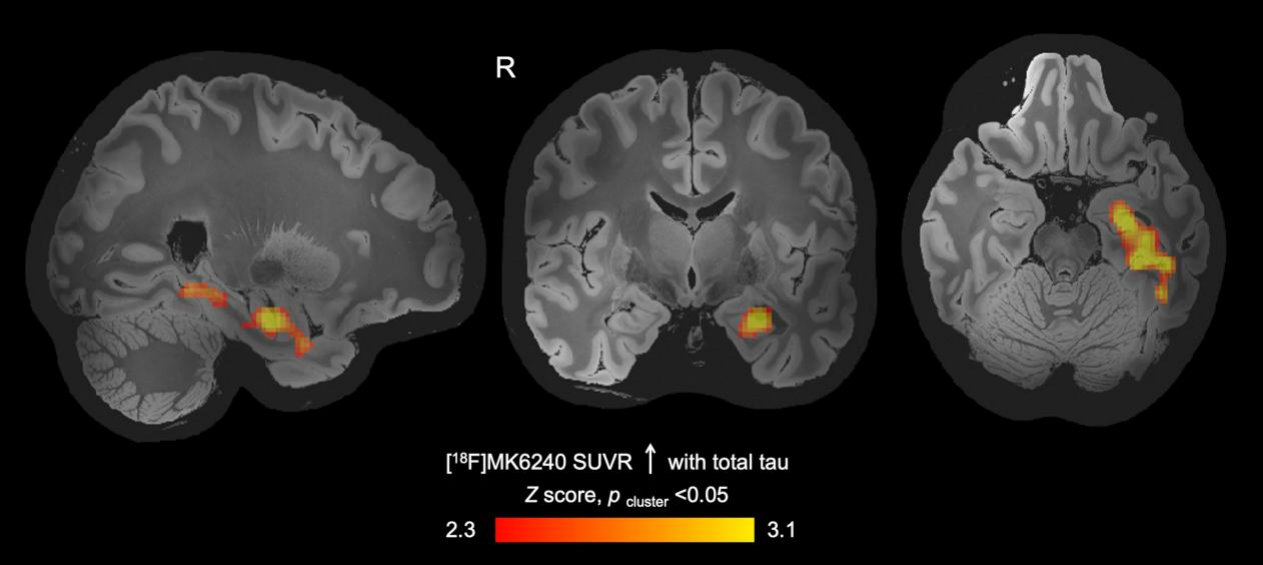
Relationship between brain PHF tau measured by [^18^F]MK6240 PET and blood total tau in active-duty SOF. Blood total tau levels were positively associated with PHF as measured by [^18^F]MK6240 SUVR in a region encompassing the left temporal cortex, left parahippocampal gyrus and left hippocampus. Image shows a statistical map of linear regression with total tau and [^18^F]MK6240 SUVR, controlling for age [threshold of *Z* > 2.3, beta = 1.23 × 10^−2^, 95% CI (1.22 × 10^−2^, 1.23 × 10^−2^), *P_cluster_* < 0.05], overlaid on a 500-micron quantitative multi-echo flash template in MNI space.^20^ R = right, SUVR = standardized uptake value ratio, PHF = paired helical filament.
